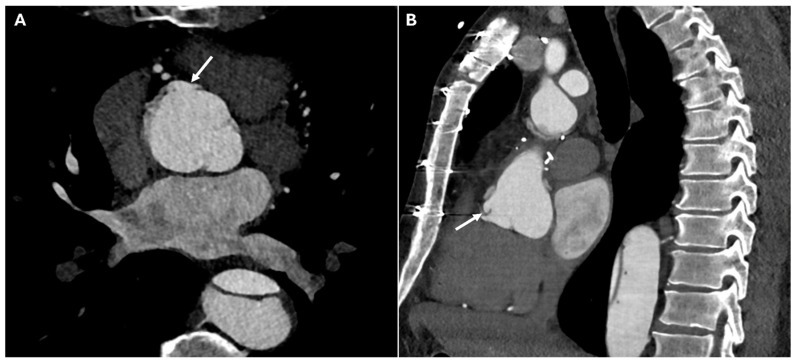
CT images of pseudoaneurysm at the proximal anastomotic site. Panels (A,B) highlight the presence of a localized, contained pseudoaneurysm in the vessel wall at the site where the proximal graft is sutured to the ascending aorta, characterized by an outpouching of surrounding blood (white arrows).
